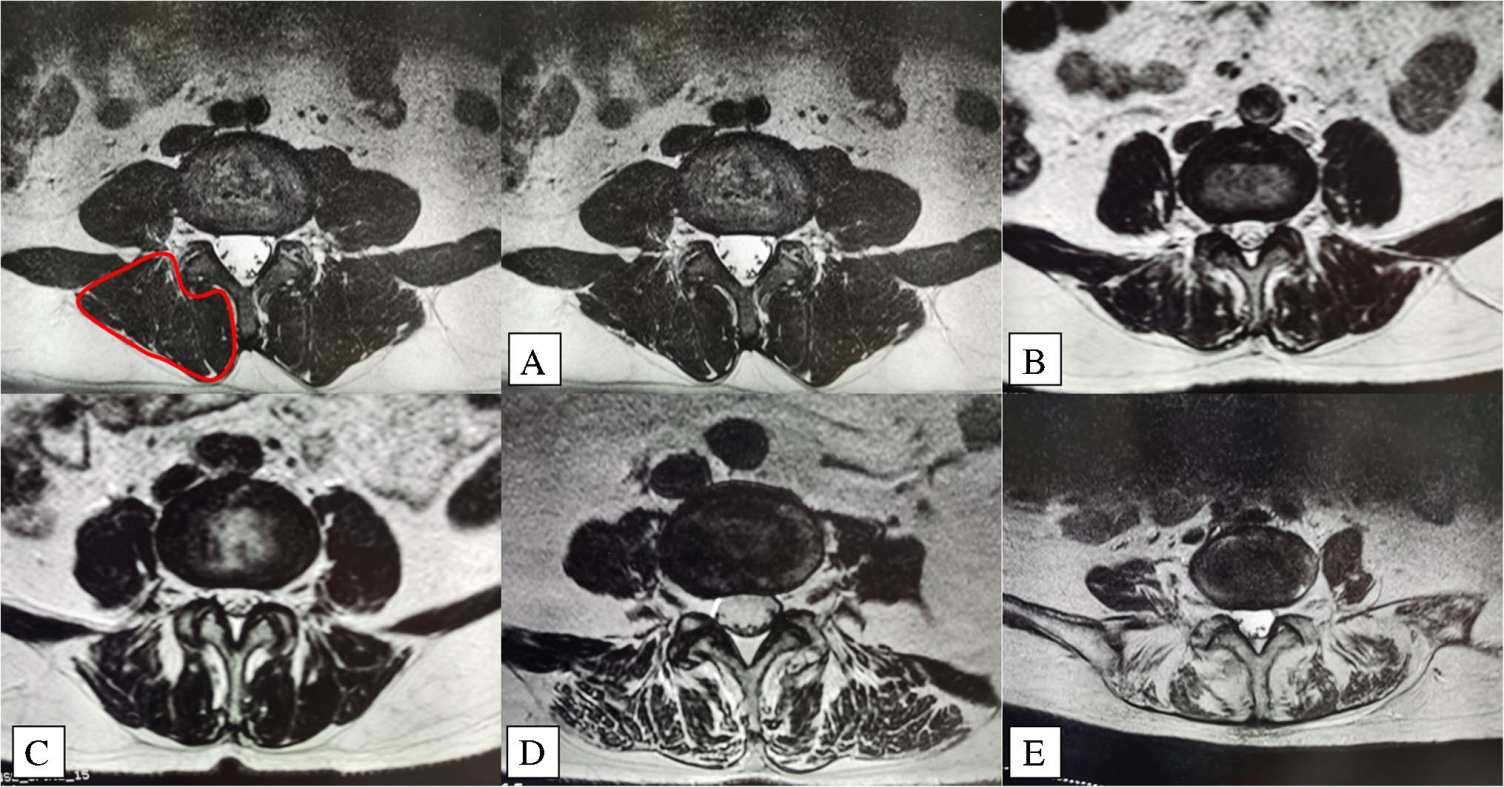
Goutallier classification. Fascial boundary of the paraspinal muscle (red line) on axial T2-weighted MRI. A Grade 0, normal, almost no fat infiltration in the muscle. B Grade 1, fatty streaks within the muscle. C Grade 2, fat infiltration was less than muscle mass. D Grade 3, fat infiltration was approximately equal to muscle mass. E Grade 4, fat infiltration was greater than muscle mass
